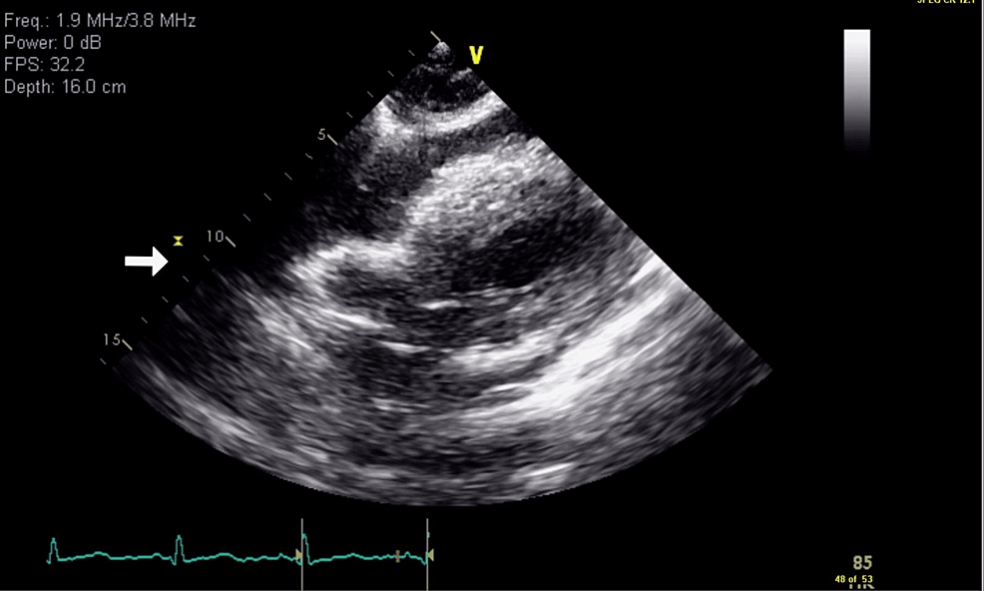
Transthoracic echocardiogram showing cardiac tamponade
